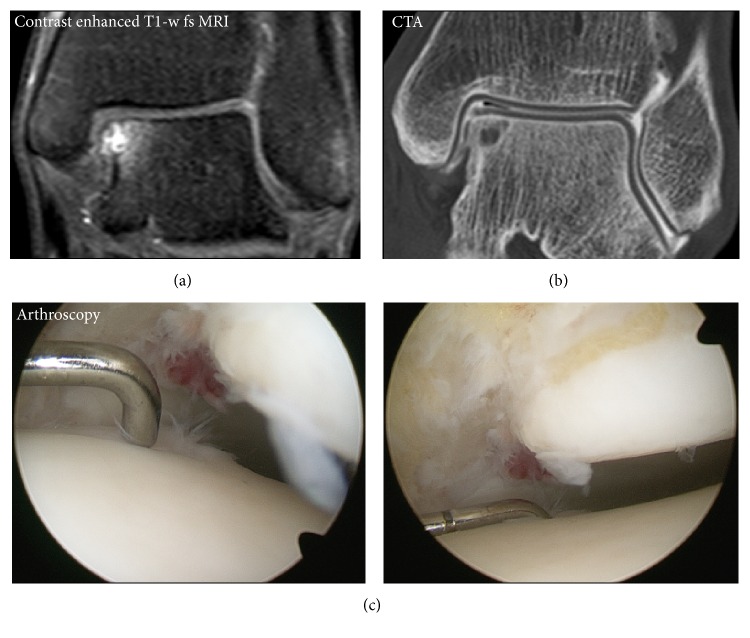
Considering intraoperative findings as standard of reference. CTA showed improved sensitivity compared to MRI. Exemplarily, in this subject with an osteochondral defect at the medial talus only BMEL but no cartilage defect was depicted on MRI (a). The full thickness cartilage defect revealed by CTA (b) was confirmed with a probe intraoperatively (c).
